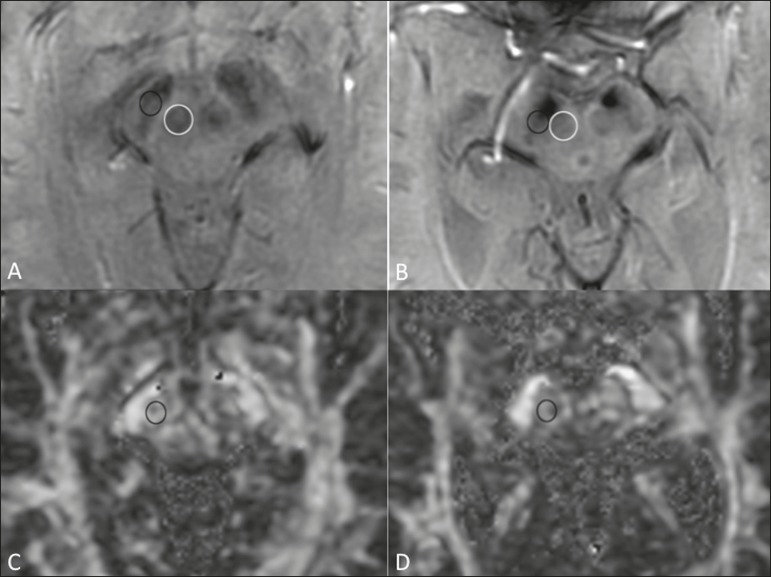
Findings on conventional MRI and DTI in Parkinson's disease. On
